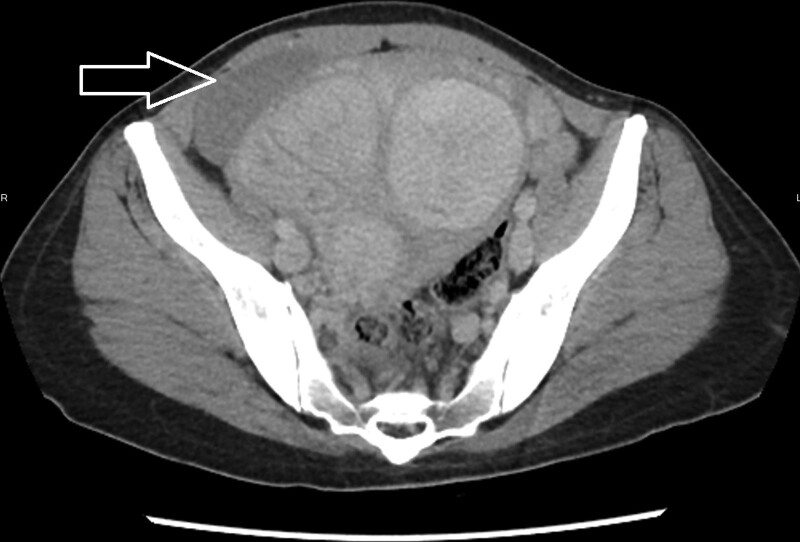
Axial view of CT imaging with contrast enhancement showed a poor contrast enhancement subserosal leiomyoma on right uterine surface (arrow). CT = computed tomography.
